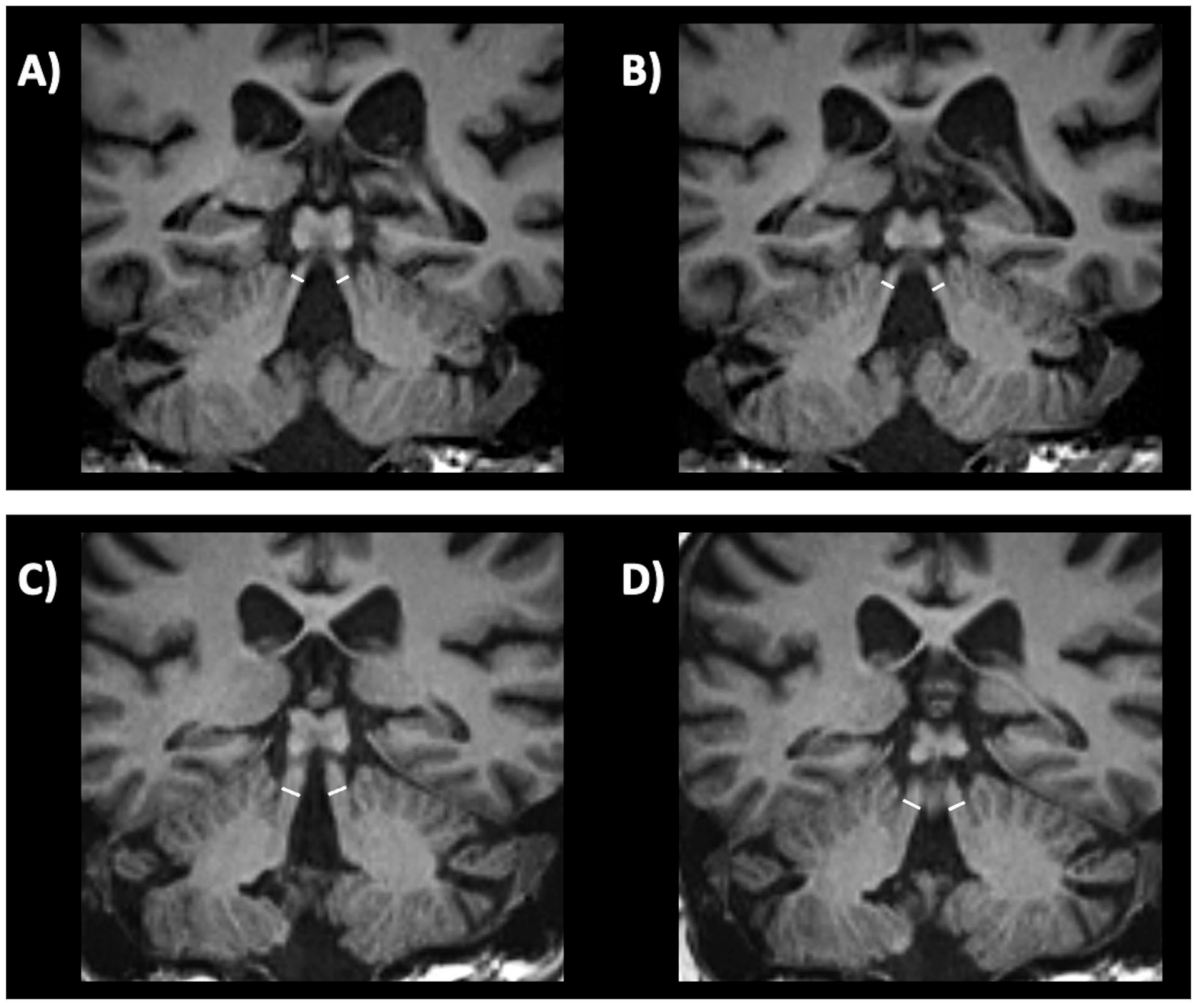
Three-tesla brain T1-weighted oblique coronal MR images parallel to the floor of the fourth ventricle, showing how to measure the superior cerebellar peduncle width, in a patient with progressive supranuclear palsy (A,B) and in a patient with Parkinson’s disease (C,D). This structure is more atrophic in progressive supranuclear palsy than in Parkinson’s disease.
